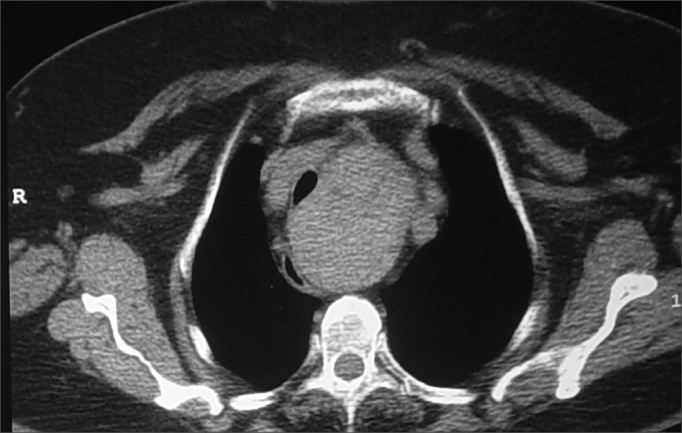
CT scan showing goiter displacing mediastinal structures.
